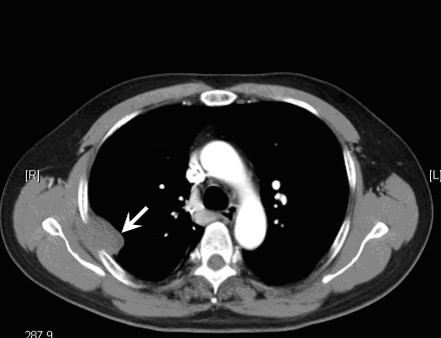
Chest computed tomography showing lung metastasis with pleural seeding 80 months after hepatectomy.
